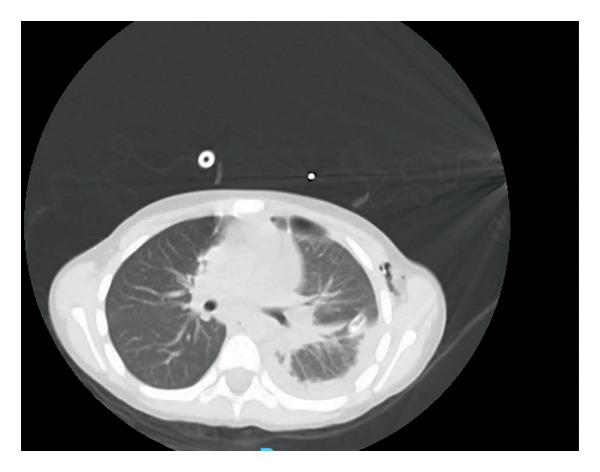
Axial noncontrast CT scan prior to discharge demonstrating interval progression of cavitation within the left lower lobe following segmentectomy, with a small loculated pneumothorax. The left pleural effusion has significantly decreased.
